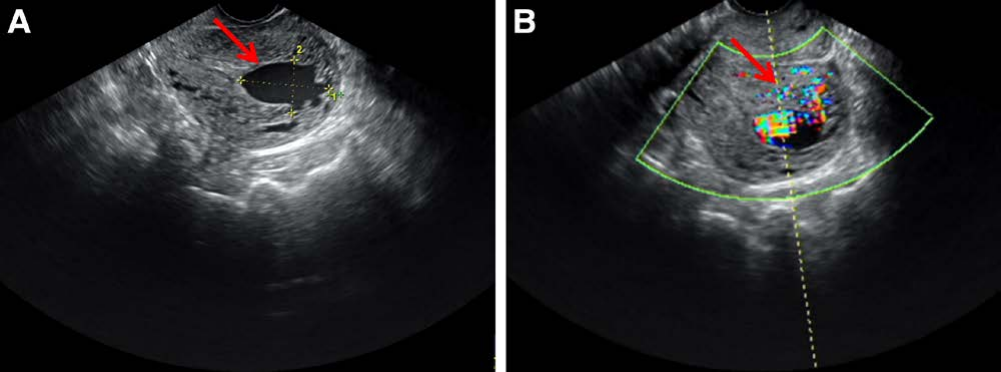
Ultrasonographic features of a uterine arteriovenous malformation. (A) Separation of the upper middle segment of the uterine cavity suggested a cystic dark area measuring 3.1 * 1.9 cm (arrow). (B) Pulsed-wave Doppler shows a mixed arteriovenous flow pattern.
